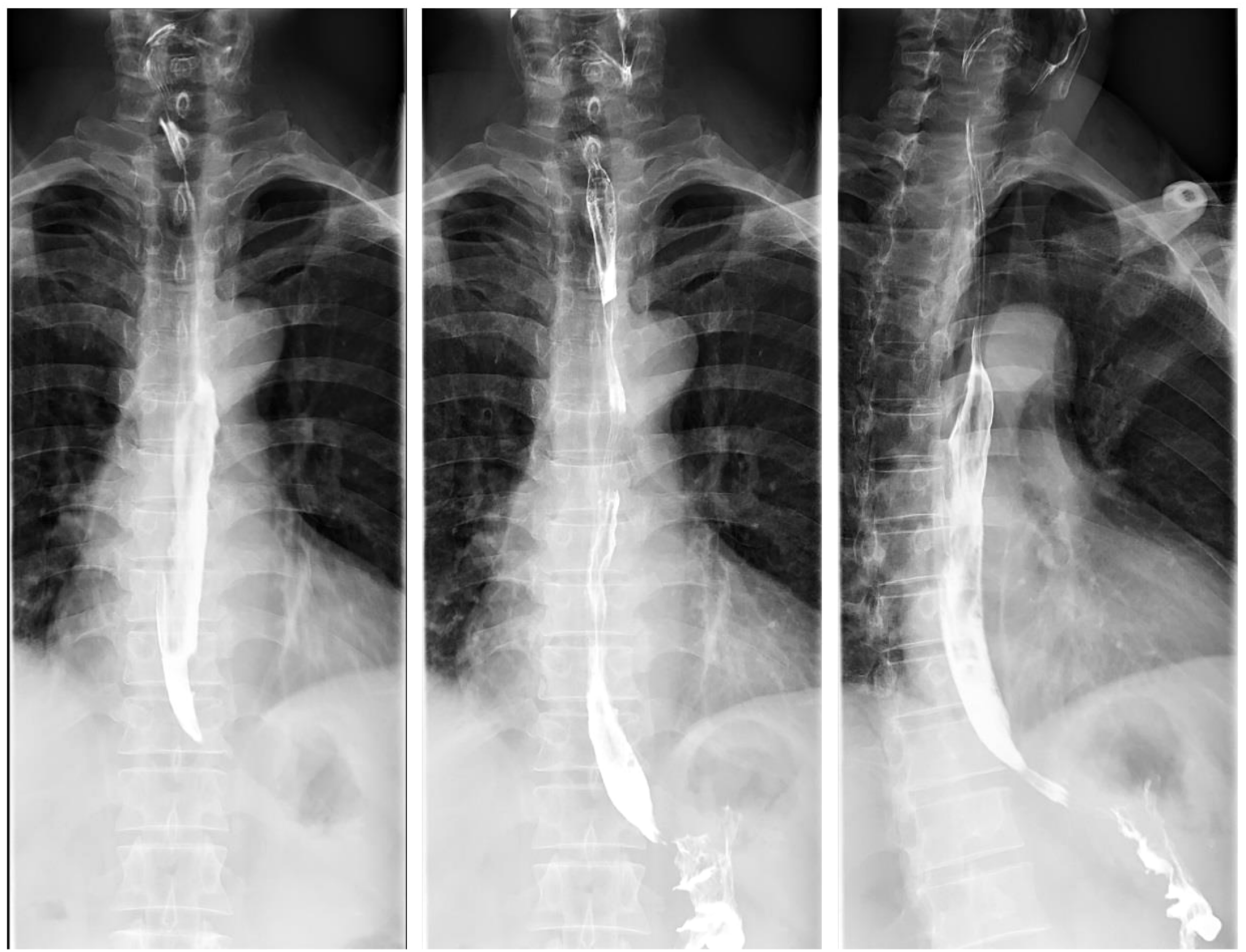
Barium meal radiography of the esophagus showing esophageal lesions at our hospital.
